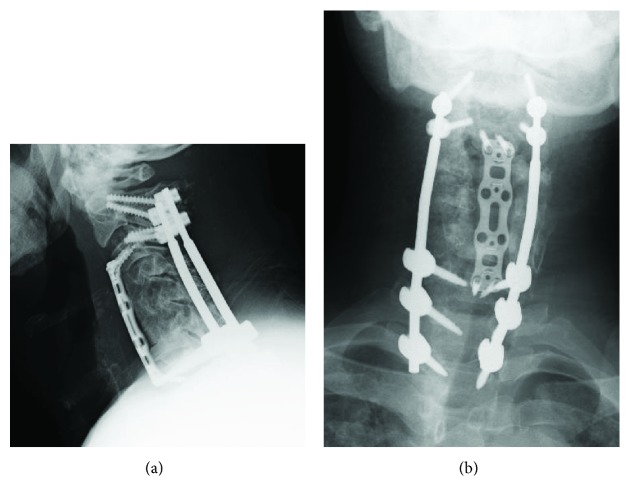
X-ray of the cervical spine obtained just after the two-stage additional surgery showed restoration of the cervical alignment by posterior and anterior spinal fusion surgery.
